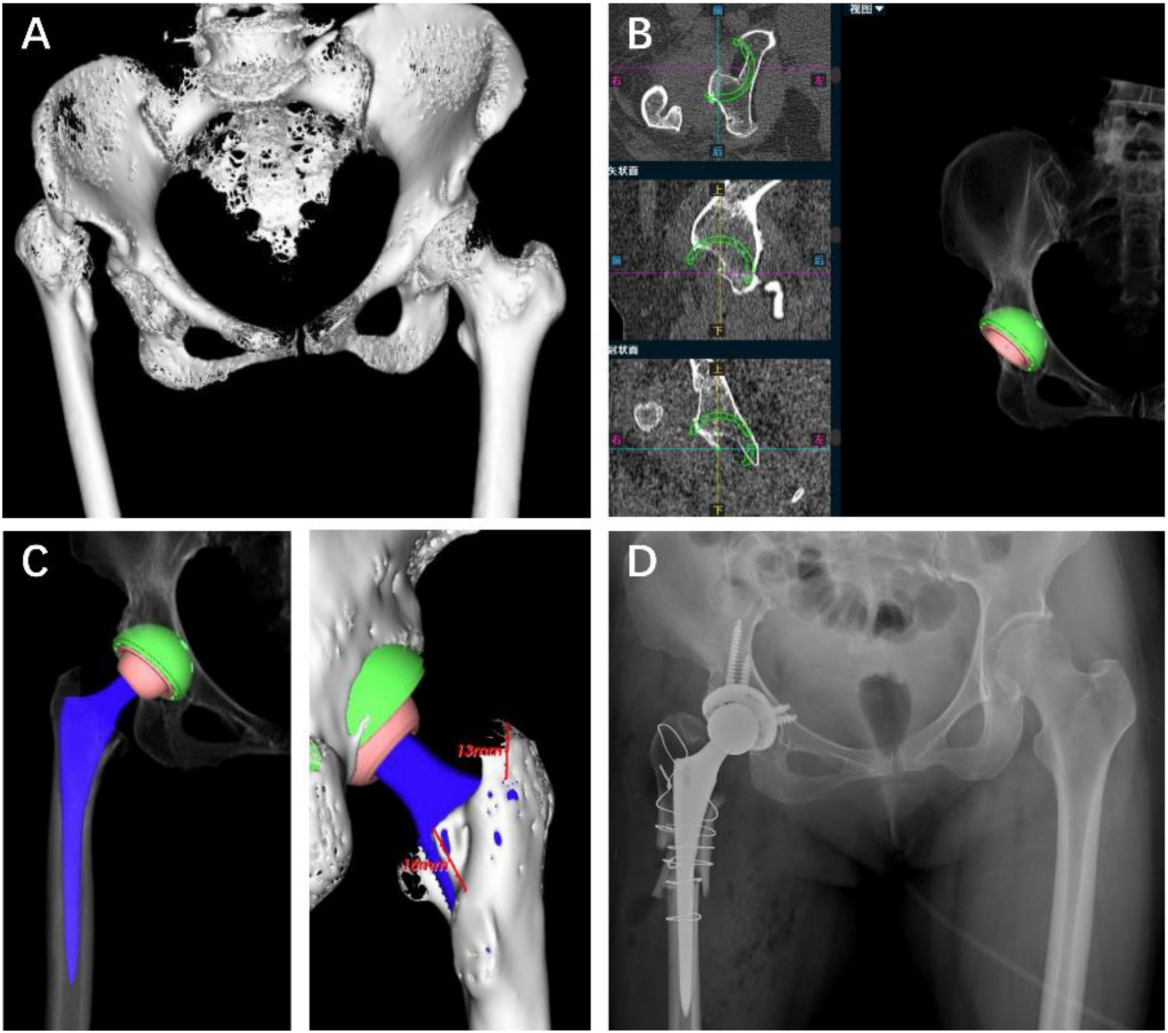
AI-assisted 3D preoperative planning in a Crowe IV post-septic HOA case. (A) 3D CT reconstruction. (B) Planned acetabular cup (Pinnacle, size 44). (C) Planned femoral stem (Summit, size 1), with the template exceeding the femoral bony envelope. (D) Postoperative radiograph showing the final position of the planned implants.
